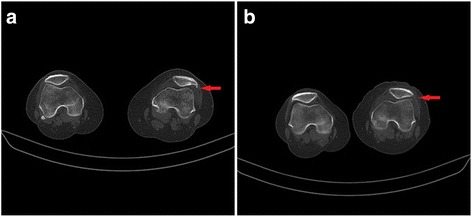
Typical case I: female, 41 years old, pain for 48 months. a Preoperatively, Lysholm score 74, CA 25°, PTA 10°, and PLS 9 mm; CT scan showed Wiberg IV patella. b After the operation on the left knee, Lysholm score 75, CA 1°, PTA 9°, and PLS 8 mm; CT scan showed approximate Wiberg II patella
